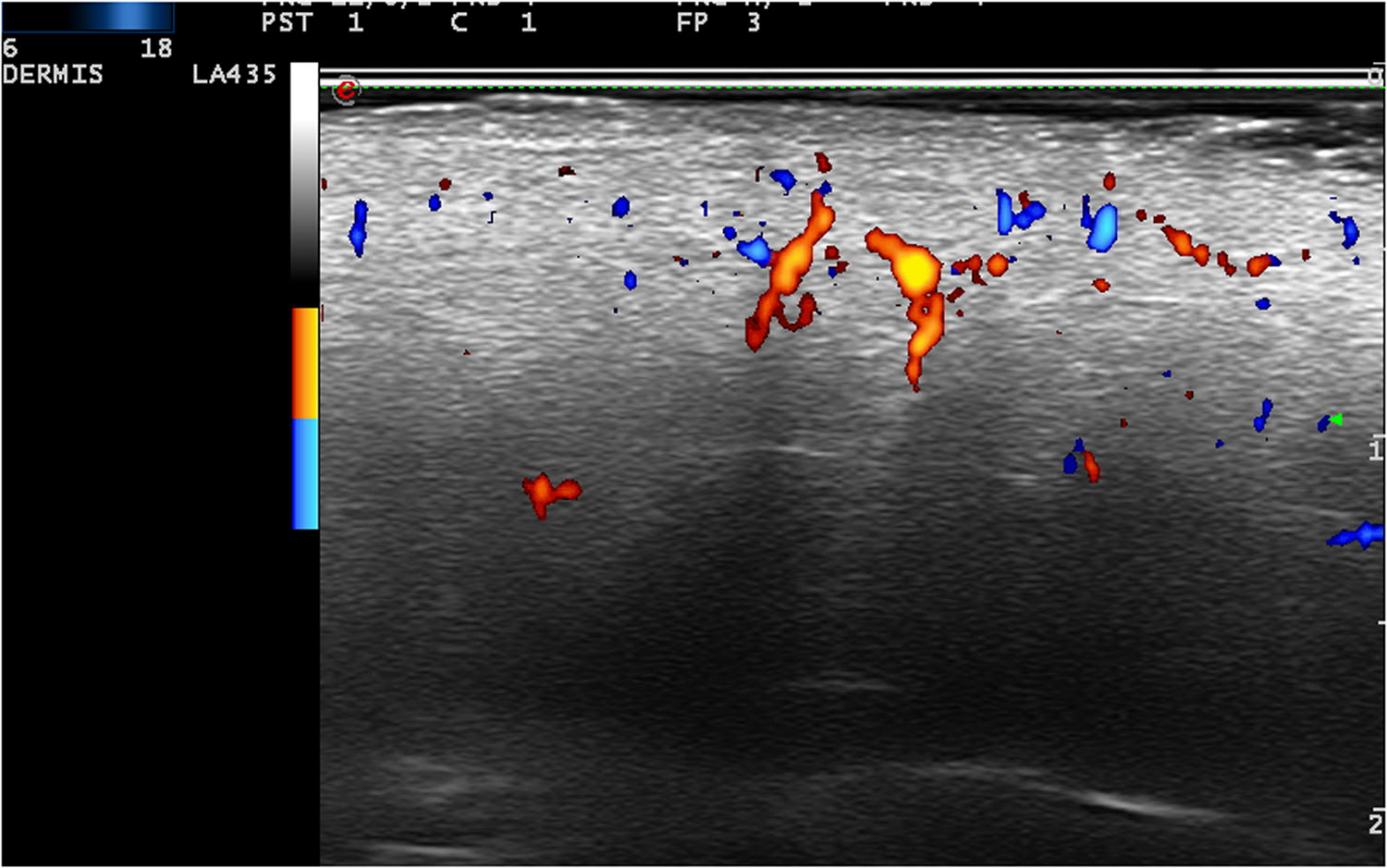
Case 5. Power Doppler Colour: increased vascularisation in dermis and hypodermis.
